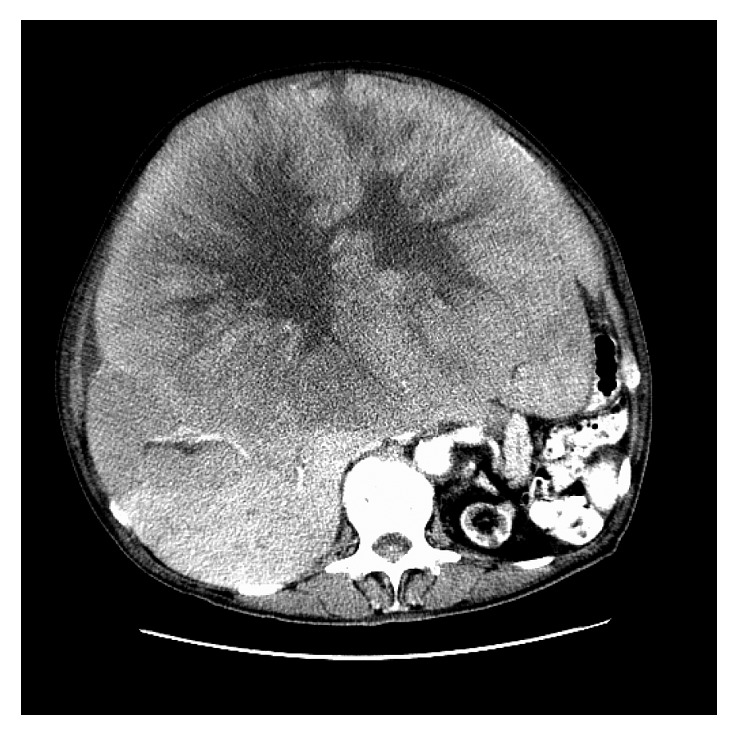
Abdominal CT scan at diagnosis.
